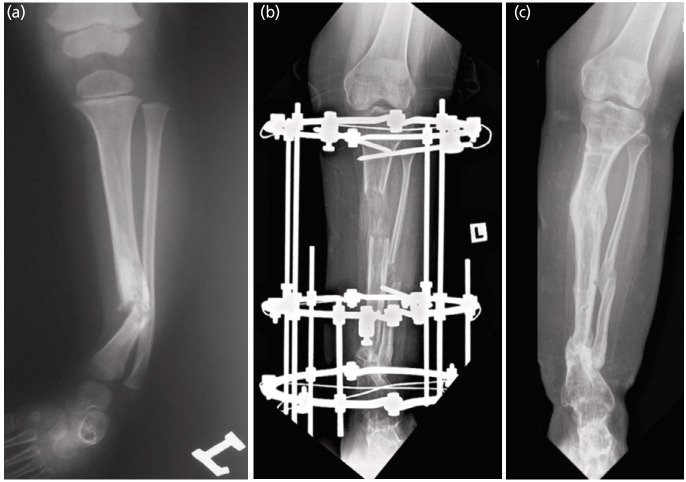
Tibial radiographs of Case 7. She had four surgical procedures since the age of 2 years, refractured twice and achieved union at age 17 years. (a) Congenital pseudoarthrosis of the tibia at age one year. (b) IEF lengthening at age 17 years. (c) Age 19 years with 4cm LLD and 24o distal tibial valgus – Johnston Grade II.
